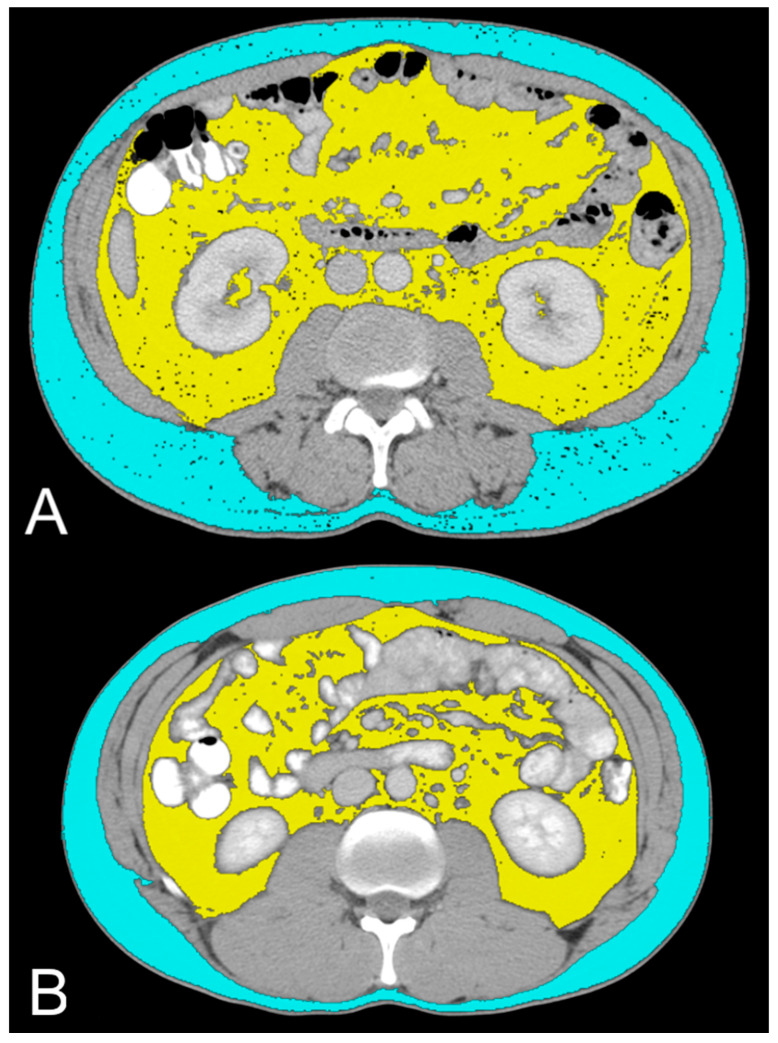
Axial CT images showing VAT segmented in yellow and SAT segmented in light blue in low-grade ccRCC patients with ADFP expression (A) and high-Fuhrman-grade ccRCC patients with ADFP expression (B).
